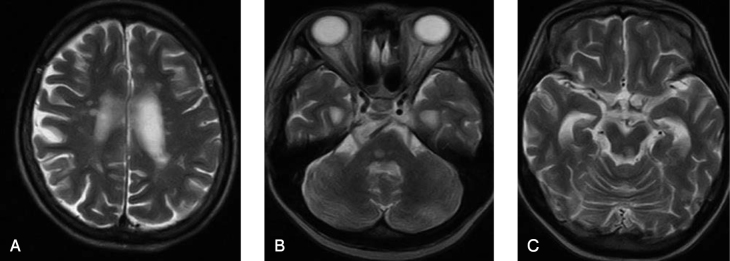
Brain MRI (T2-weighted) showed multiple newly appearing focal lesions in the subcortical area (2A), hyperintensity in the left optic nerve (2 B), and in the left midbrain tegmentium (2C) at 47 years of age.
